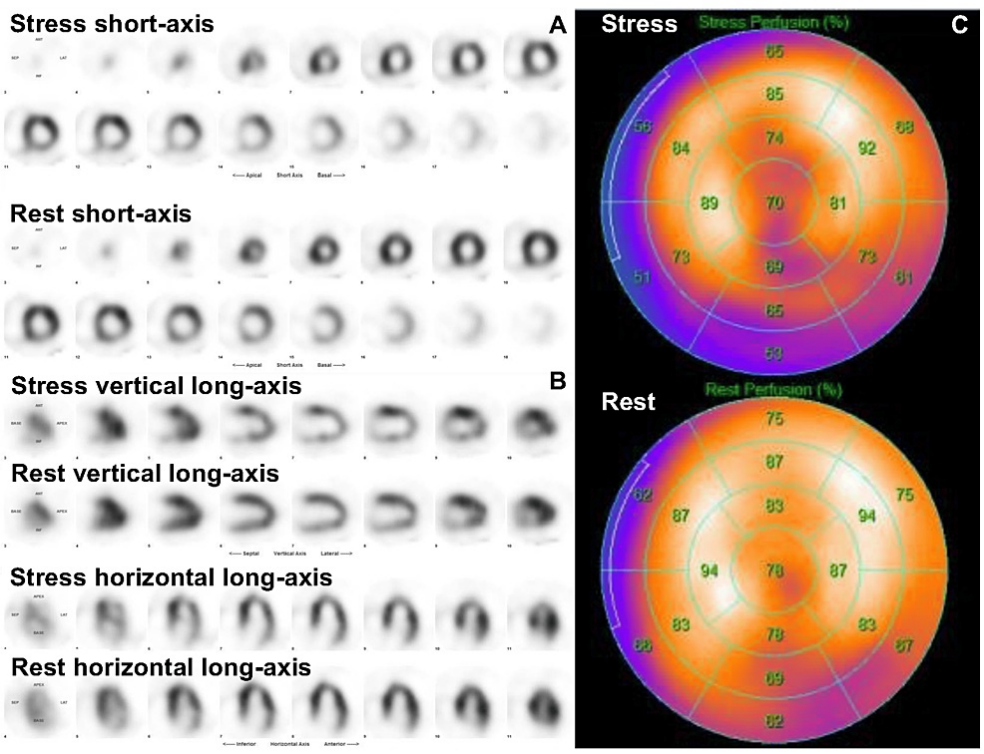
Results of myocardial perfusion scintigraphy Stress images show moderately reduced uptake in the inferior wall and mildly reduced uptake in the anterior and apex walls. Rest images show fill-in in the inferior, anterior, and apex walls. A) Short-axis images of the left ventricle at stress (the top two rows) and at rest (the bottom two rows). B) Vertical (the top two rows) and horizontal (the bottom two rows) long-axis images of the left ventricle at stress (upper) and at rest (lower). C) Bull’s-eye map at stress (upper) and at rest (lower).
